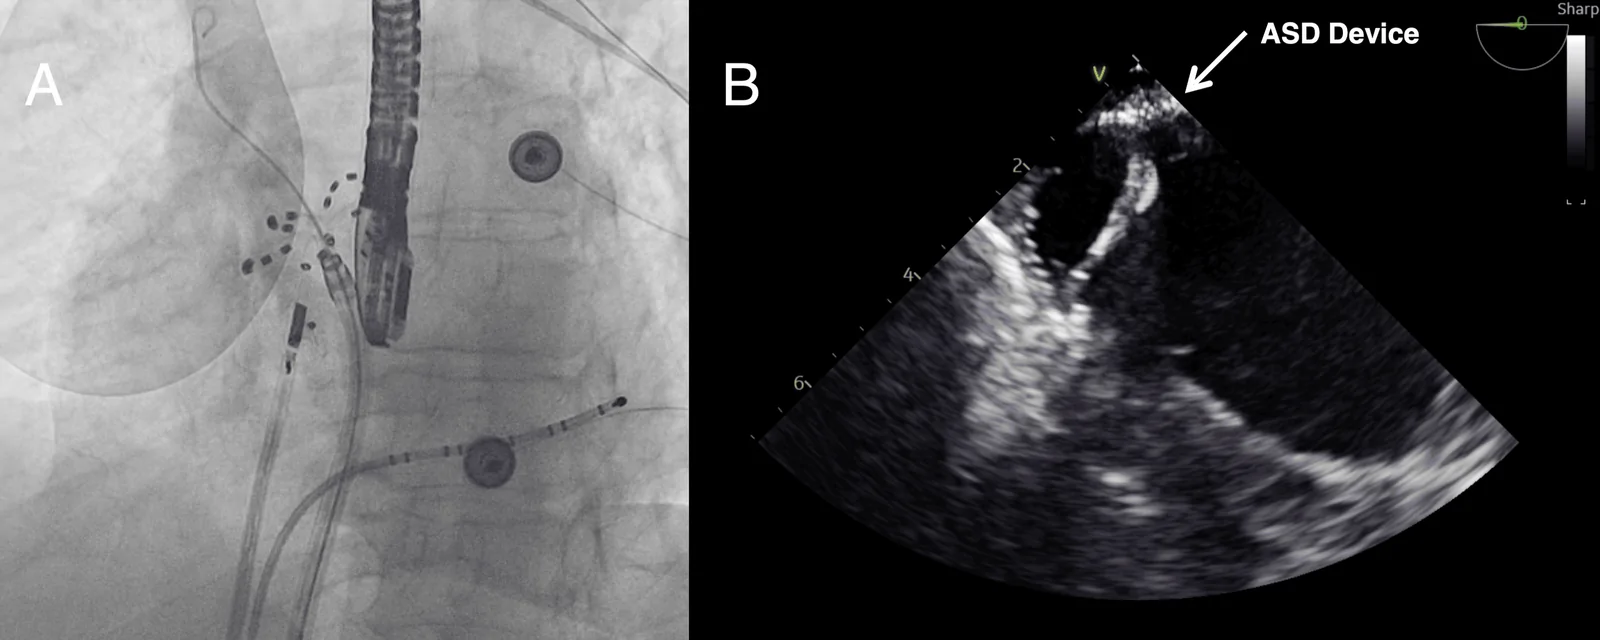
Procedural imaging (A) Fluoroscopy and (B) intracardiac echocardiography confirming the position of the Farawave ablation catheter, with care taken to avoid contact between the catheter spines and the atrial septal defect (ASD) closure device.
